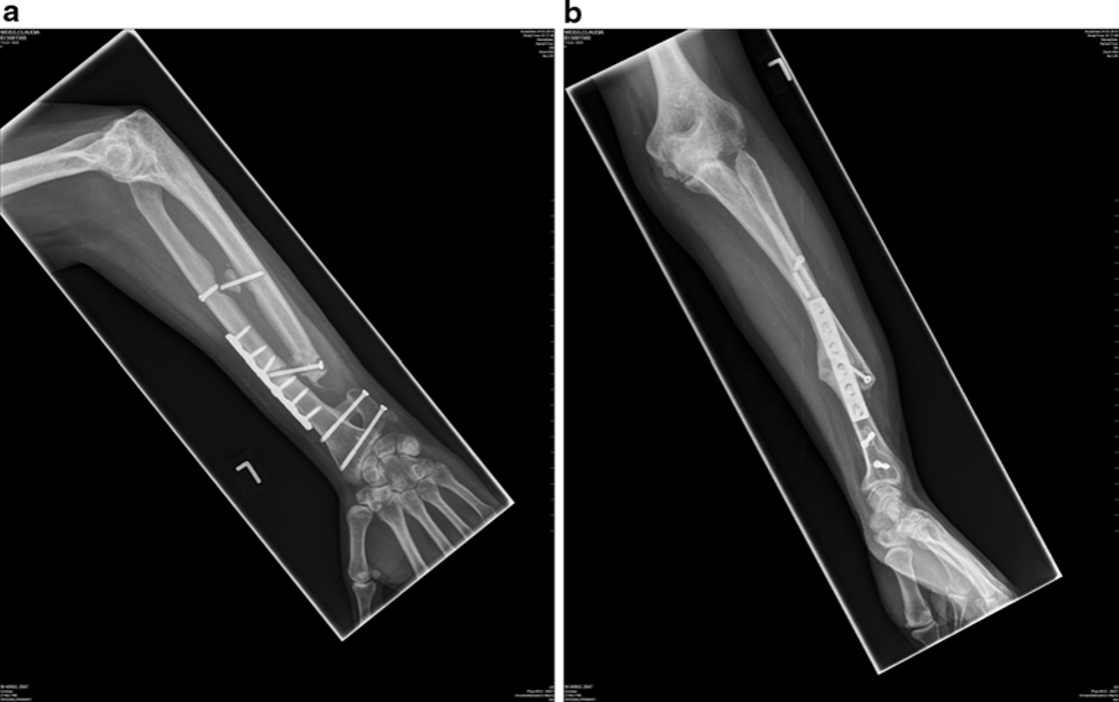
AP and lateral forearm radiographs showing failed synostosis
